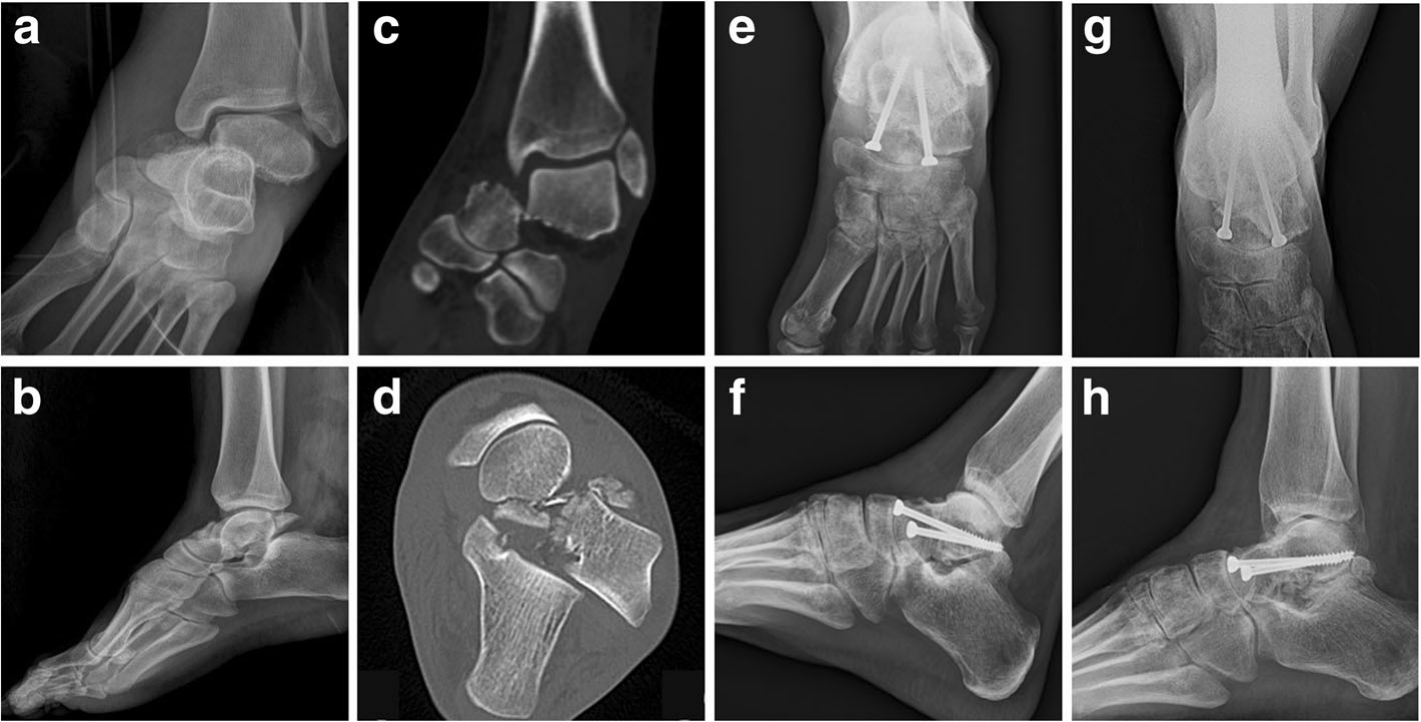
Case 2: a 41-year-old woman with a Hawkins type IV fracture presenting signs of union despite a disastrous initial condition. Antero-posterior and lateral radiographic images at preoperative period (a-b), coronal and axial CT images at preoperative period (c-d), antero-posterior and lateral radiographic images at immediate postoperative period (e-f), antero-posterior and lateral radiographic images at 51-month follow-up (g-h)
